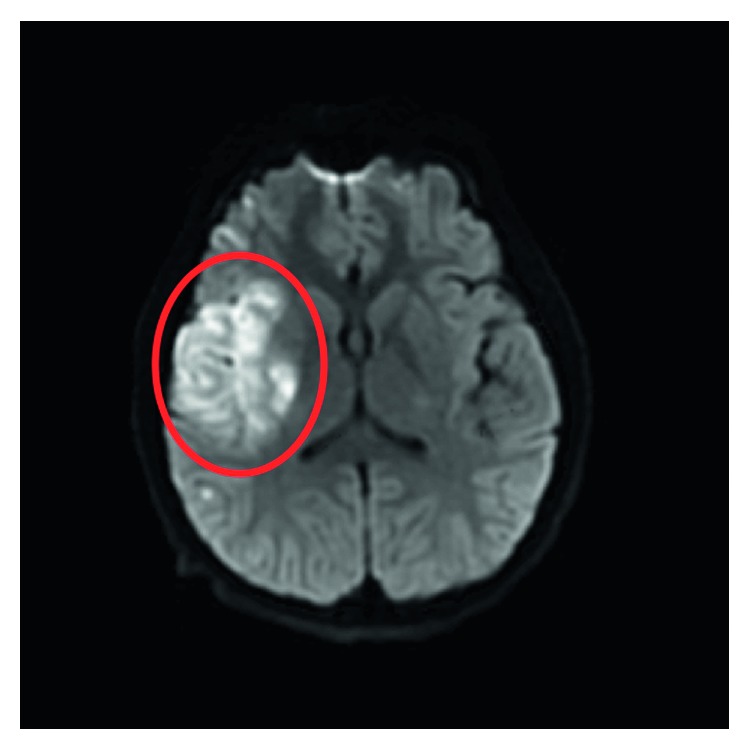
Diffusion weighted imaging (DWI) sequence demonstrating restricted diffusion in the right MCA territory of the basal ganglia and temporal lobe.
